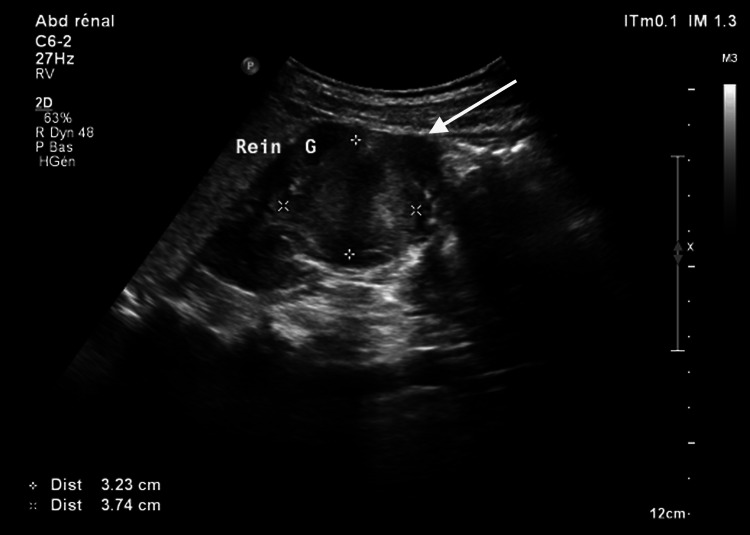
Longitudinal ultrasound image of the left kidney showing a well-defined isoechoic cortical mass extending into the renal sinus, measuring approximately 3.2 × 3.7 cm (white arrow)
